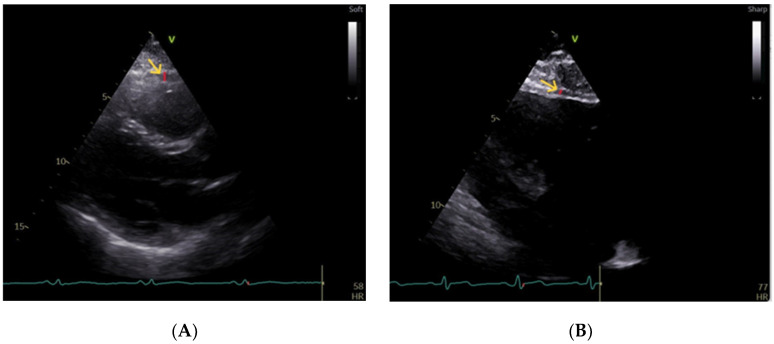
Epicardial fat thickness measurement by echocardiography in the parasternal longitudinal view (green arrow indicating the area of interest). Epicardial fat (indicated by red line and yellow arrow) with increased thickness in a patient with OSA (A) and minimum epicardial fat in a healthy volunteer (B).
